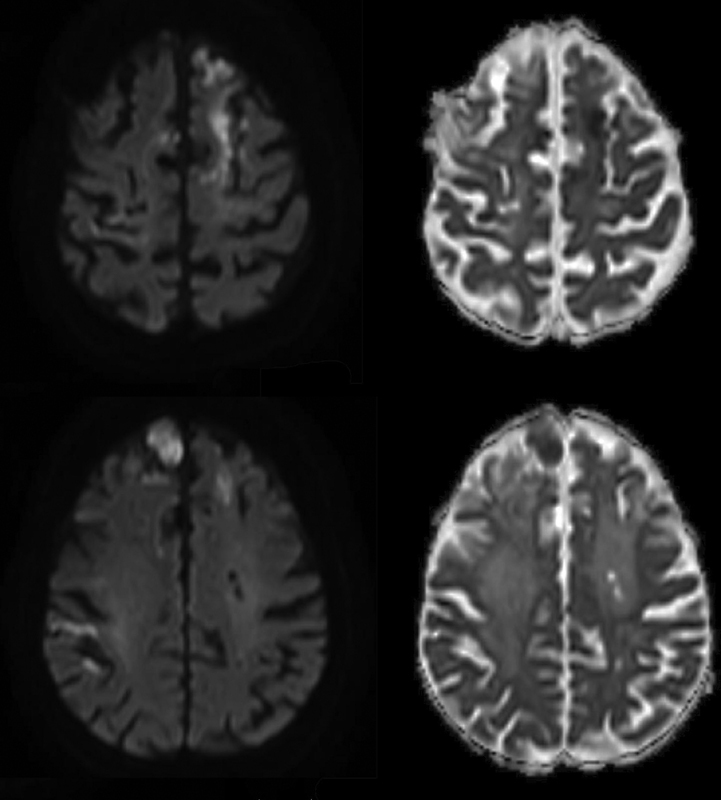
MRI on the left side diffusion-weighted imaging (DWI), on the right side apparent diffusion coefficient (ADC) showing multiple bilateral infarcts of various age as a result of progressive disease in the course of 1 year and 8 months after the first hospital admission.
